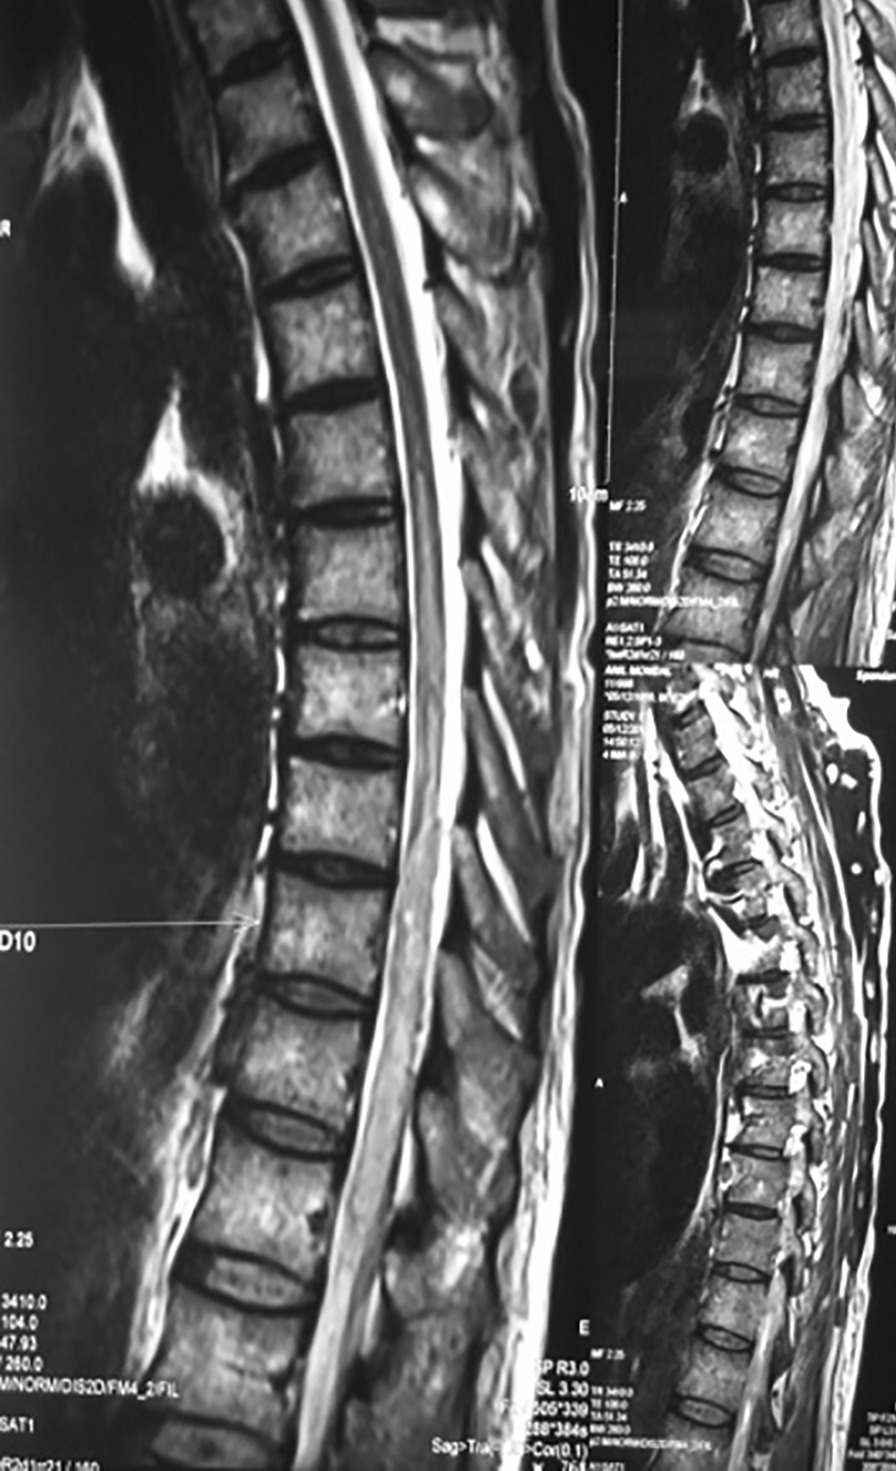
Magnetic Resonance Imaging of dorsal spine (plain and contrast) showing diffuse cord swelling with edema in the lower dorsal spinal cord extending up to conus medullaris accompanied by patchy enhancement within. Extensive neurocysticercosis within both paravertebral as well as posterior paraspinal muscles is visible. (Arrow shows the position of 10th thoracic vertebra)
